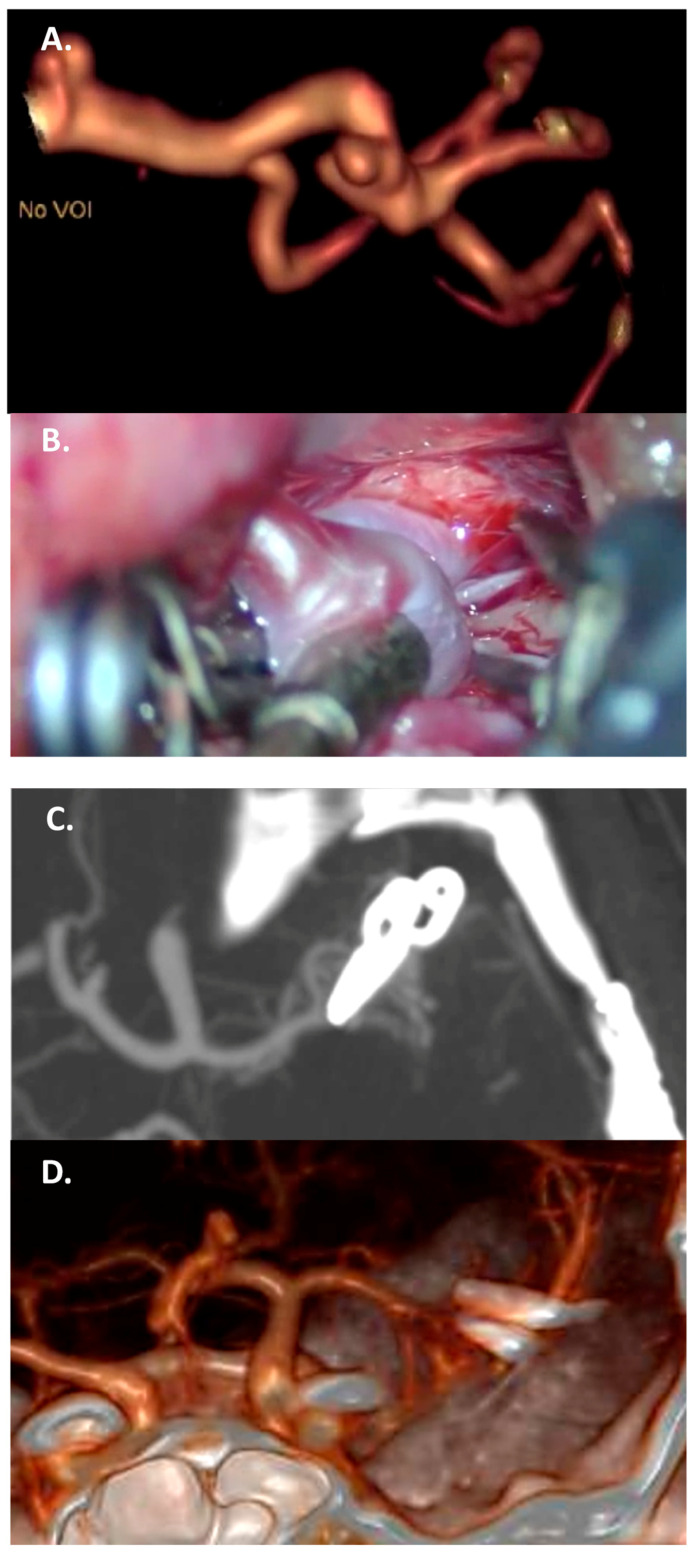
Case 2: complex clipping in a fusiform 7 mm M2 aneurysm with vessel branching from the dome, in a 35-year-old woman. (A) Preoperative CTA with a 3D reconstruction. (B) Intraoperative view of two tandem clips used to reconstruct the parent vessel. (C,D) Complete exclusion of the sac, documented by the postoperative CTA, with a 3D reconstruction.
